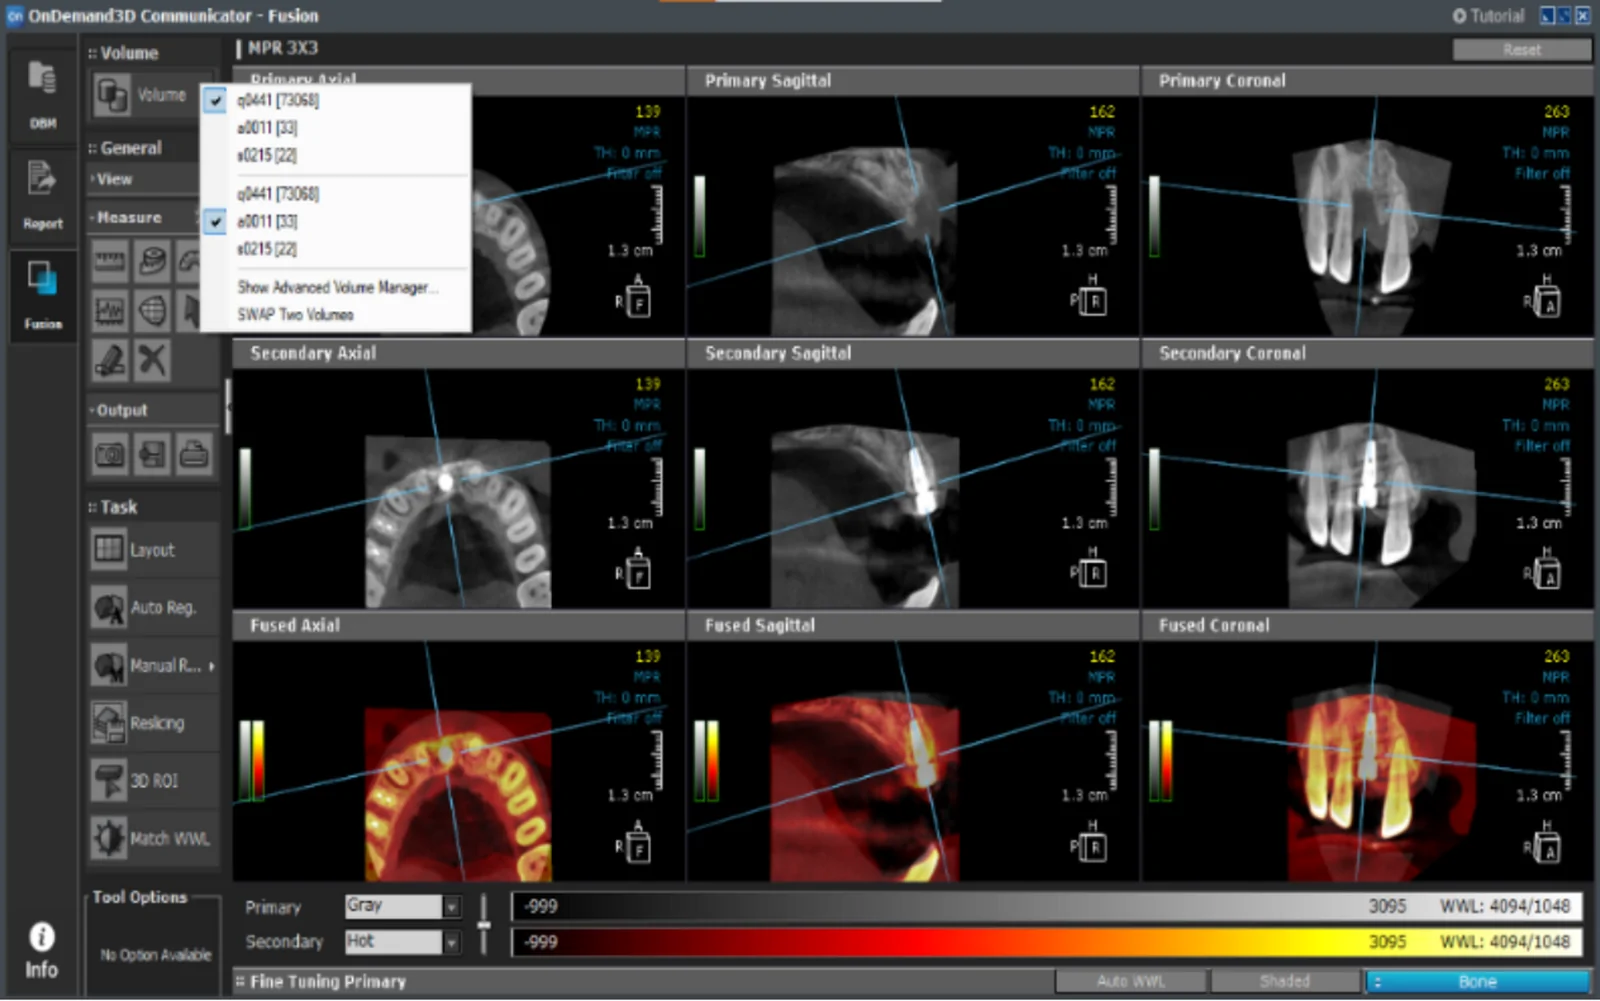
The three fused CBCT datasets
